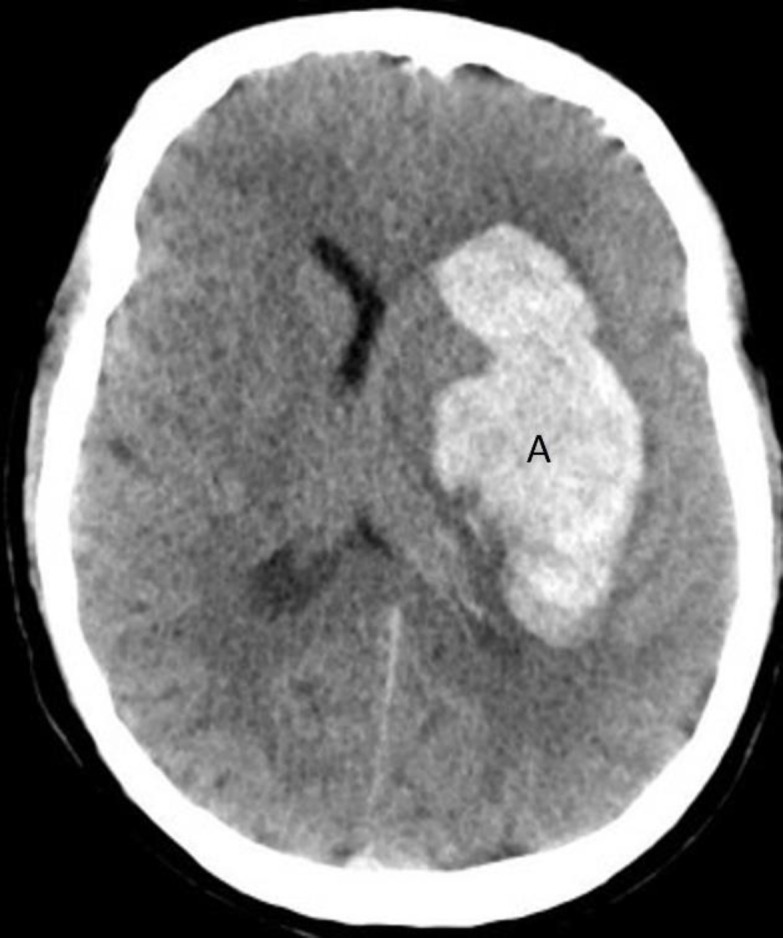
Non-contrast axial CT brain of a 51 years-old female with uncontrolled hypertension shows an intracerebral hemorrhage involving the left basal ganglia (A). Note the compression of the ipsilateral ventricle and midline shift
